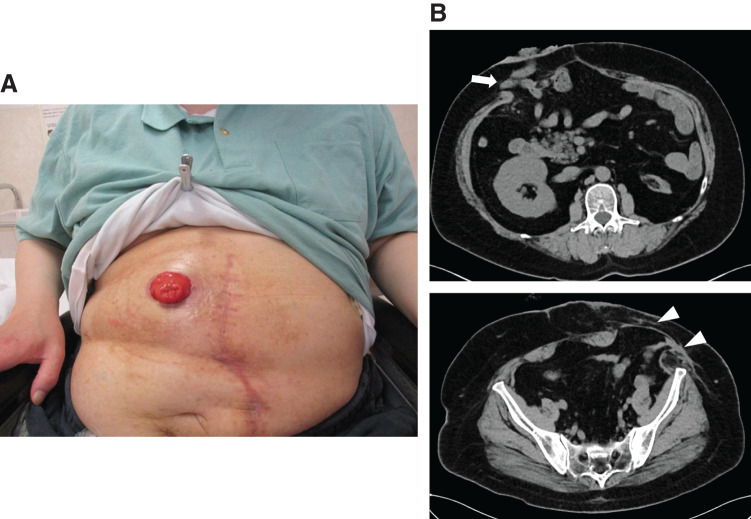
Follow-up. (A) A physical examination 6 months later shows that the abdominal wall is flat, making stoma management easier. (B) A plain abdominal CT shows a small hernia (➡) at the stoma site, but no hernia at the former stoma site (▲).
